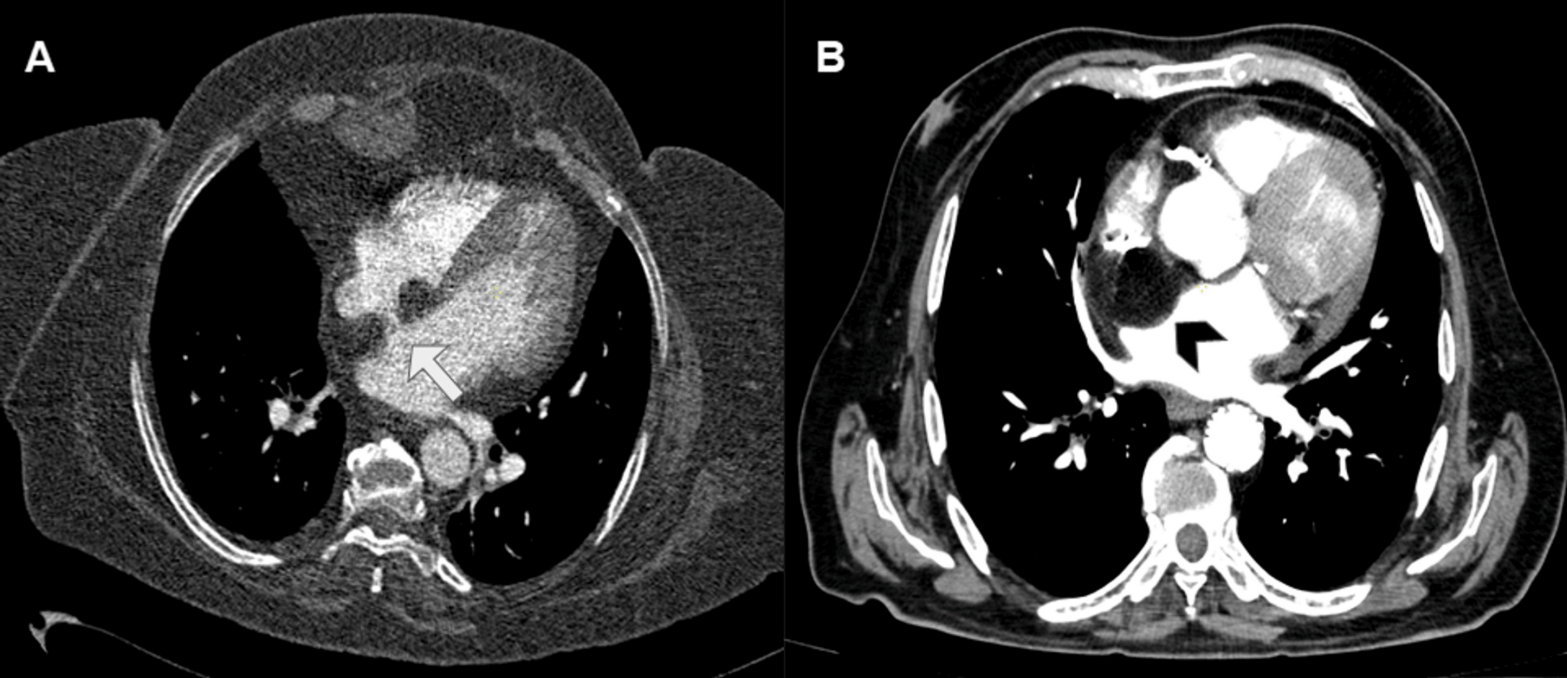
CECT in axial view in two different patients. CECT shows a lipomatous hypertrophy of the interatrial septum with a typical dumbbell or hourglass shape due to respect for the oval fossa and absence of capsule (arrow in A). Otherwise, lipomas in the interatrial septum do not spare the fossa ovalis and are encapsulated lesions, as is shown in B (arrowhead in B). CECT: contrast-enhanced computed tomography.
